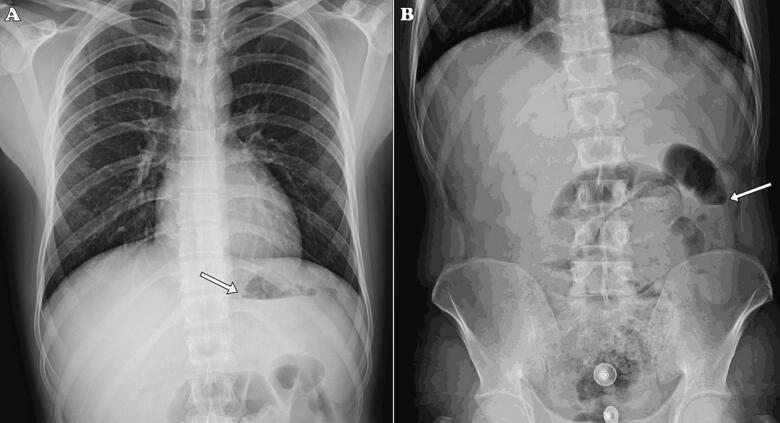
Patient's chest radiography (A) and abdominal radiography (B) with arrows indicating air-fluid levels, suggesting a potential bowel obstruction.
